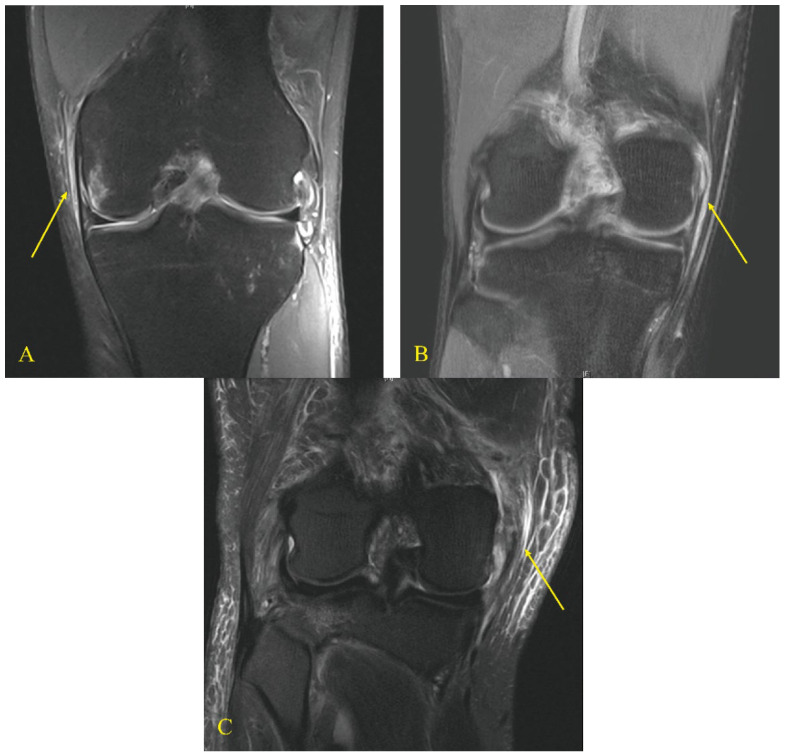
Grades of MCL injury on magnetic resonance imaging scans. Grade I (A) peri-ligamentous edema around the MCL on fluid-sensitive sequences, grade II (B) partial disruption of the ligamentous structures, and grade III (C) complete disruption of the superficial and deep MCL. Yellow arrows indicate the torn MCL based on the grade.
